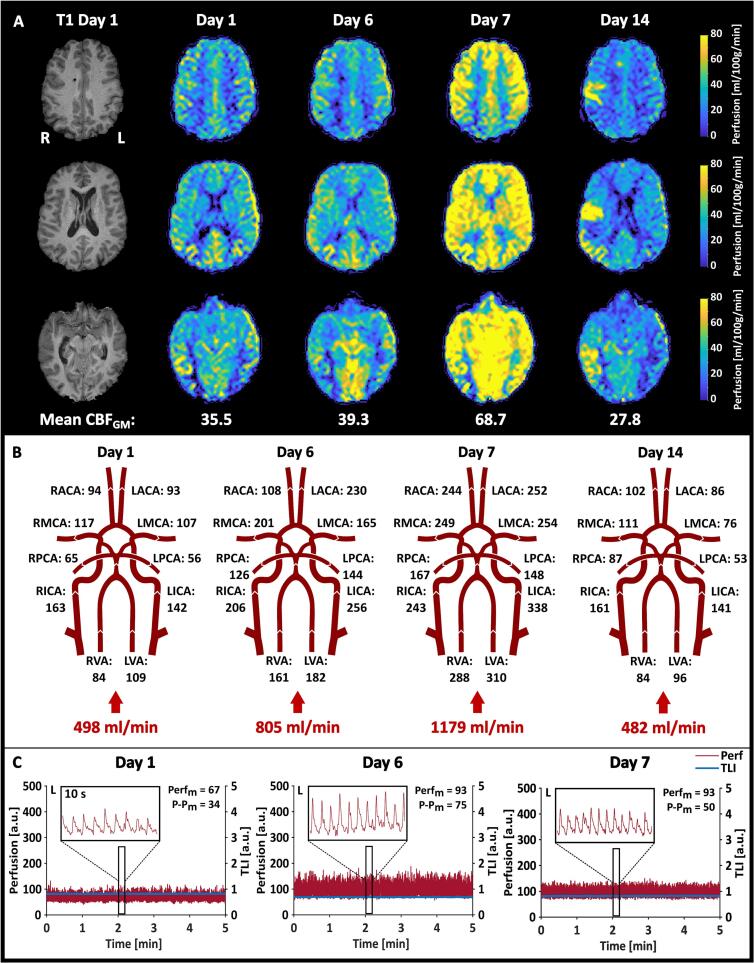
Summary of the results from the measurements performed on Patient 2. A. Absolute CBF maps calculated from the ASL data are shown in three sections. The resulting mean CBF_GM_ is depicted below each day. B. The resulting volumetric flow rates obtained from the NOVA flow data, with the calculated total inflow to the brain. C. The LDF data recorded during the 5-minute ASL MRI measurement in the left (L) hemisphere. 10-second zoomed-in sections are also depicted to show the characteristics of the perfusion signal. The resulting Perf_m_ and P-P_m_ are given in each graph.
